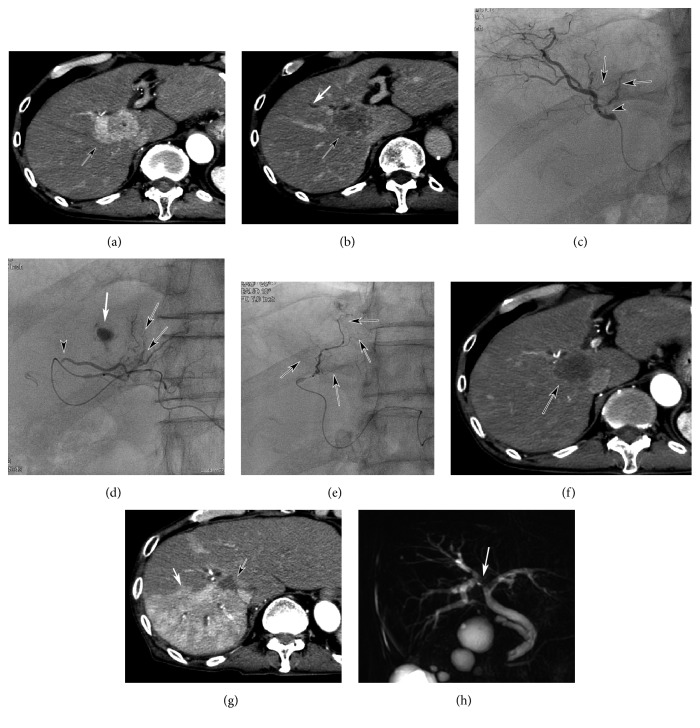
Right hepatic duct stricture complicating RAIB-TACE in a 79-year-old-patient with a HCC nodule adjacent to GS. (a) Arterial phase CT showing a hypervascular nodule (black arrow) adjacent GS. (b) Delayed phase of CT showing hypoenhancement of the tumor (black arrow) and slight biliary dilatation (white arrow) distal from the nodule. (c), (d), (e). Spot radiographies immediately after RAIB-TACE via the anterior segmental (c), caudate subsegmental (d), and medial subsegmental arteries (e). Stasis of sparse gelatin fragments with contrast medium is seen in multiple tumor feeders (black arrows, (c), (d), (f)). An anastomotic artery is seen between the caudate subsegmental (black arrowhead, (d)) and anterior segmental arteries (black arrow head, (c)). A vascular lake is also seen (white arrow, (d)). (f) CT obtained 2 months after RAIB-TACE showing CR of the tumor (black arrow). (g) CT obtained 13 months after RAIB-TACE showing shrinkage with no tumor enhancement (black arrow). The increased arterial perfusion (white arrow) to the posterior segment is due to due to cholangitis caused by stricture of the right hepatic duct. (h) MRCP showing benign stricture of the hilar bile duct (white arrow).
